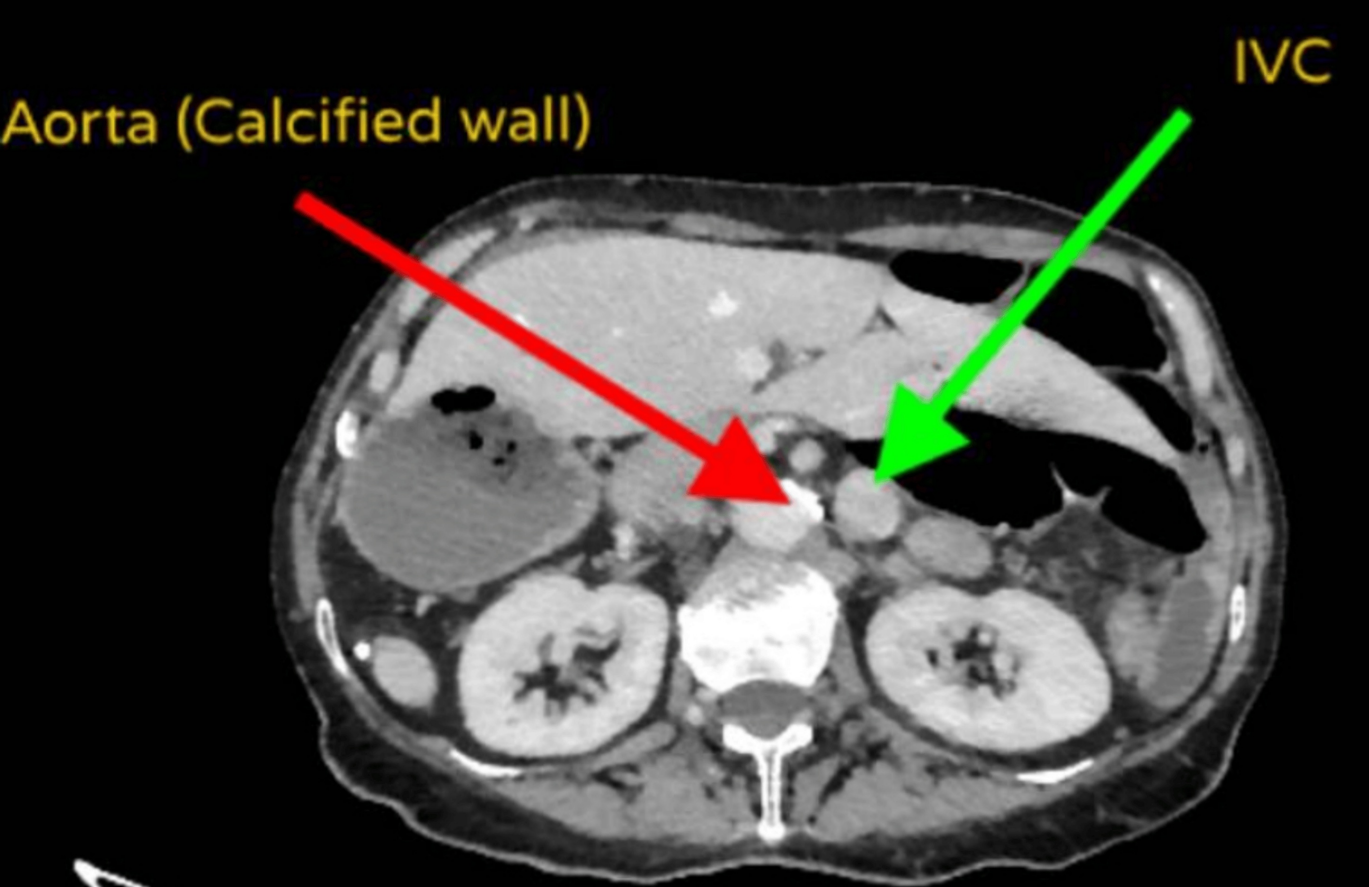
CT-TAP showing the aorta, with calcification of the aortic wall. The aorta is located on the right side and the IVC is located on the left CT-TAP: computerized tomography scan of the thorax, abdomen, and pelvis; IVC: inferior vena cava
